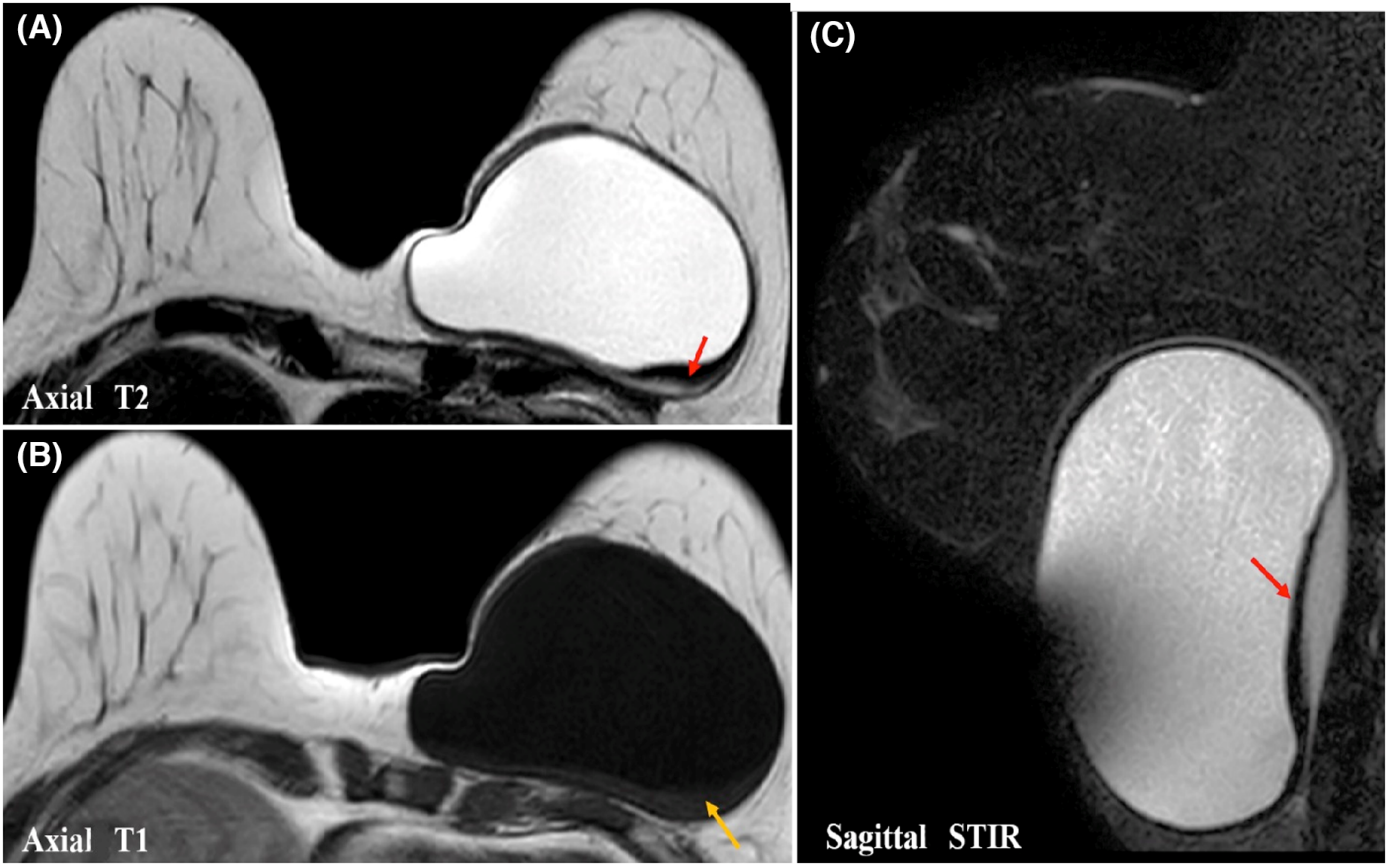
Left breast MRI: Axial T2 *(A)*, T1 *(B),* and sagittal STIR *(C)* weighted images show a cystic lesion with high signal on T2/STIR and low signal on T1. A posterior detachment of the germinal membrane which appears hypointense on T2 and STIR sequences (red arrow). The presence of a sedimentary material with intermediate T1 signal intensity is compatible with hydatid sand (yellow arrow)
